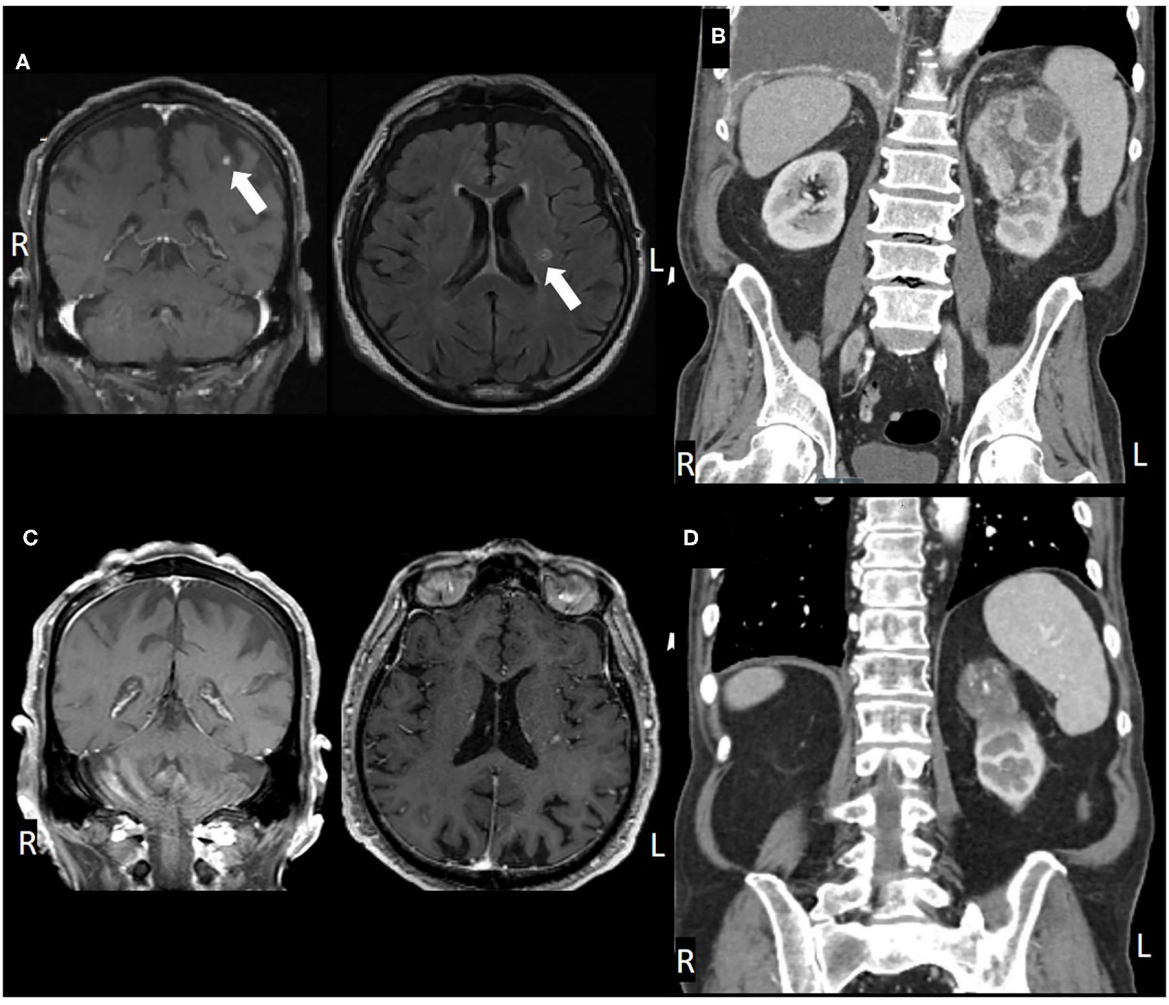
(A) Pre-treatment MRI, coronal, and axial views, (B) post-SRS and immunotherapy MRI, (C) pre-treatment CT, coronal view, and (D) post-treatment CT.
